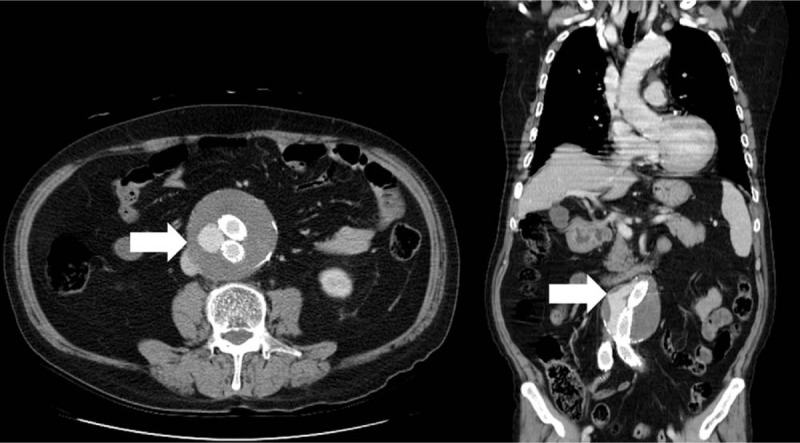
Computed tomography depicted contrast within the aneurysm sac which disclosed an endoleak (white arrow).
